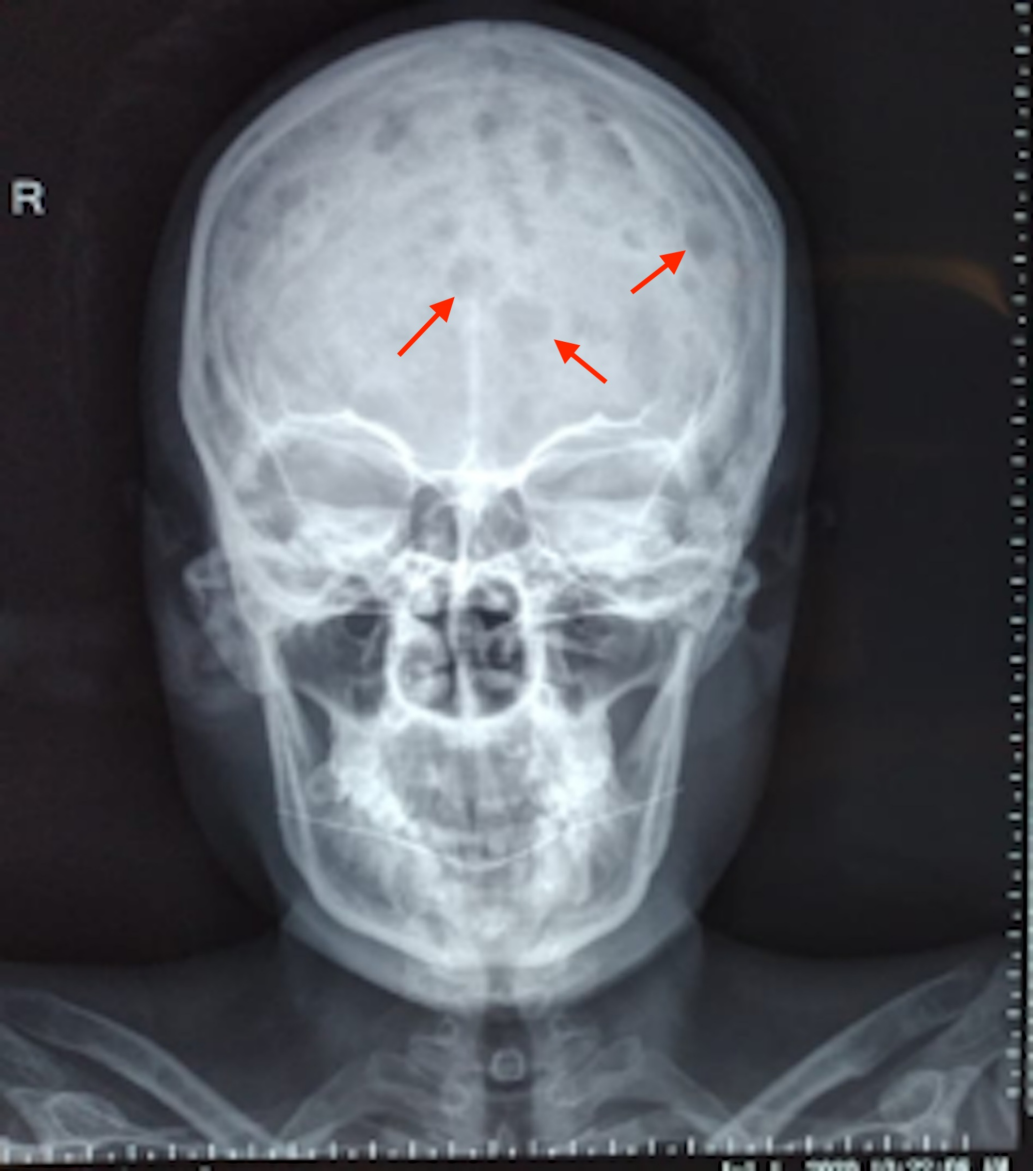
A skull radiograph depicting multiple punched out lytic lesions (arrows) indicative of multiple myeloma
